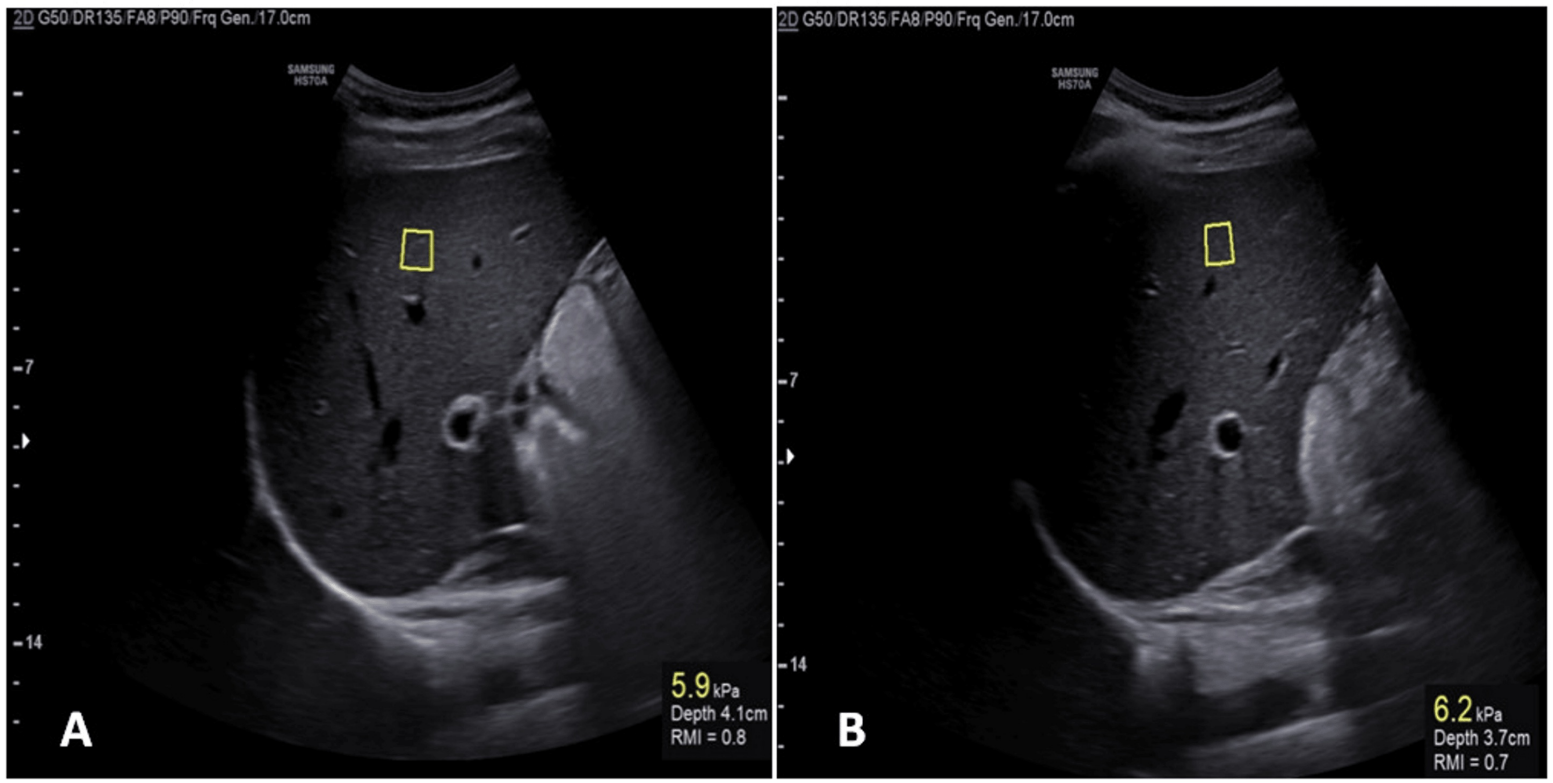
Ultrasound image utilized to assess liver stiffness. The ROI is marked by a small square (yellow box), and the liver stiffness is measured within this area ROI: region of interest; pSWE: point shear wave elastography
